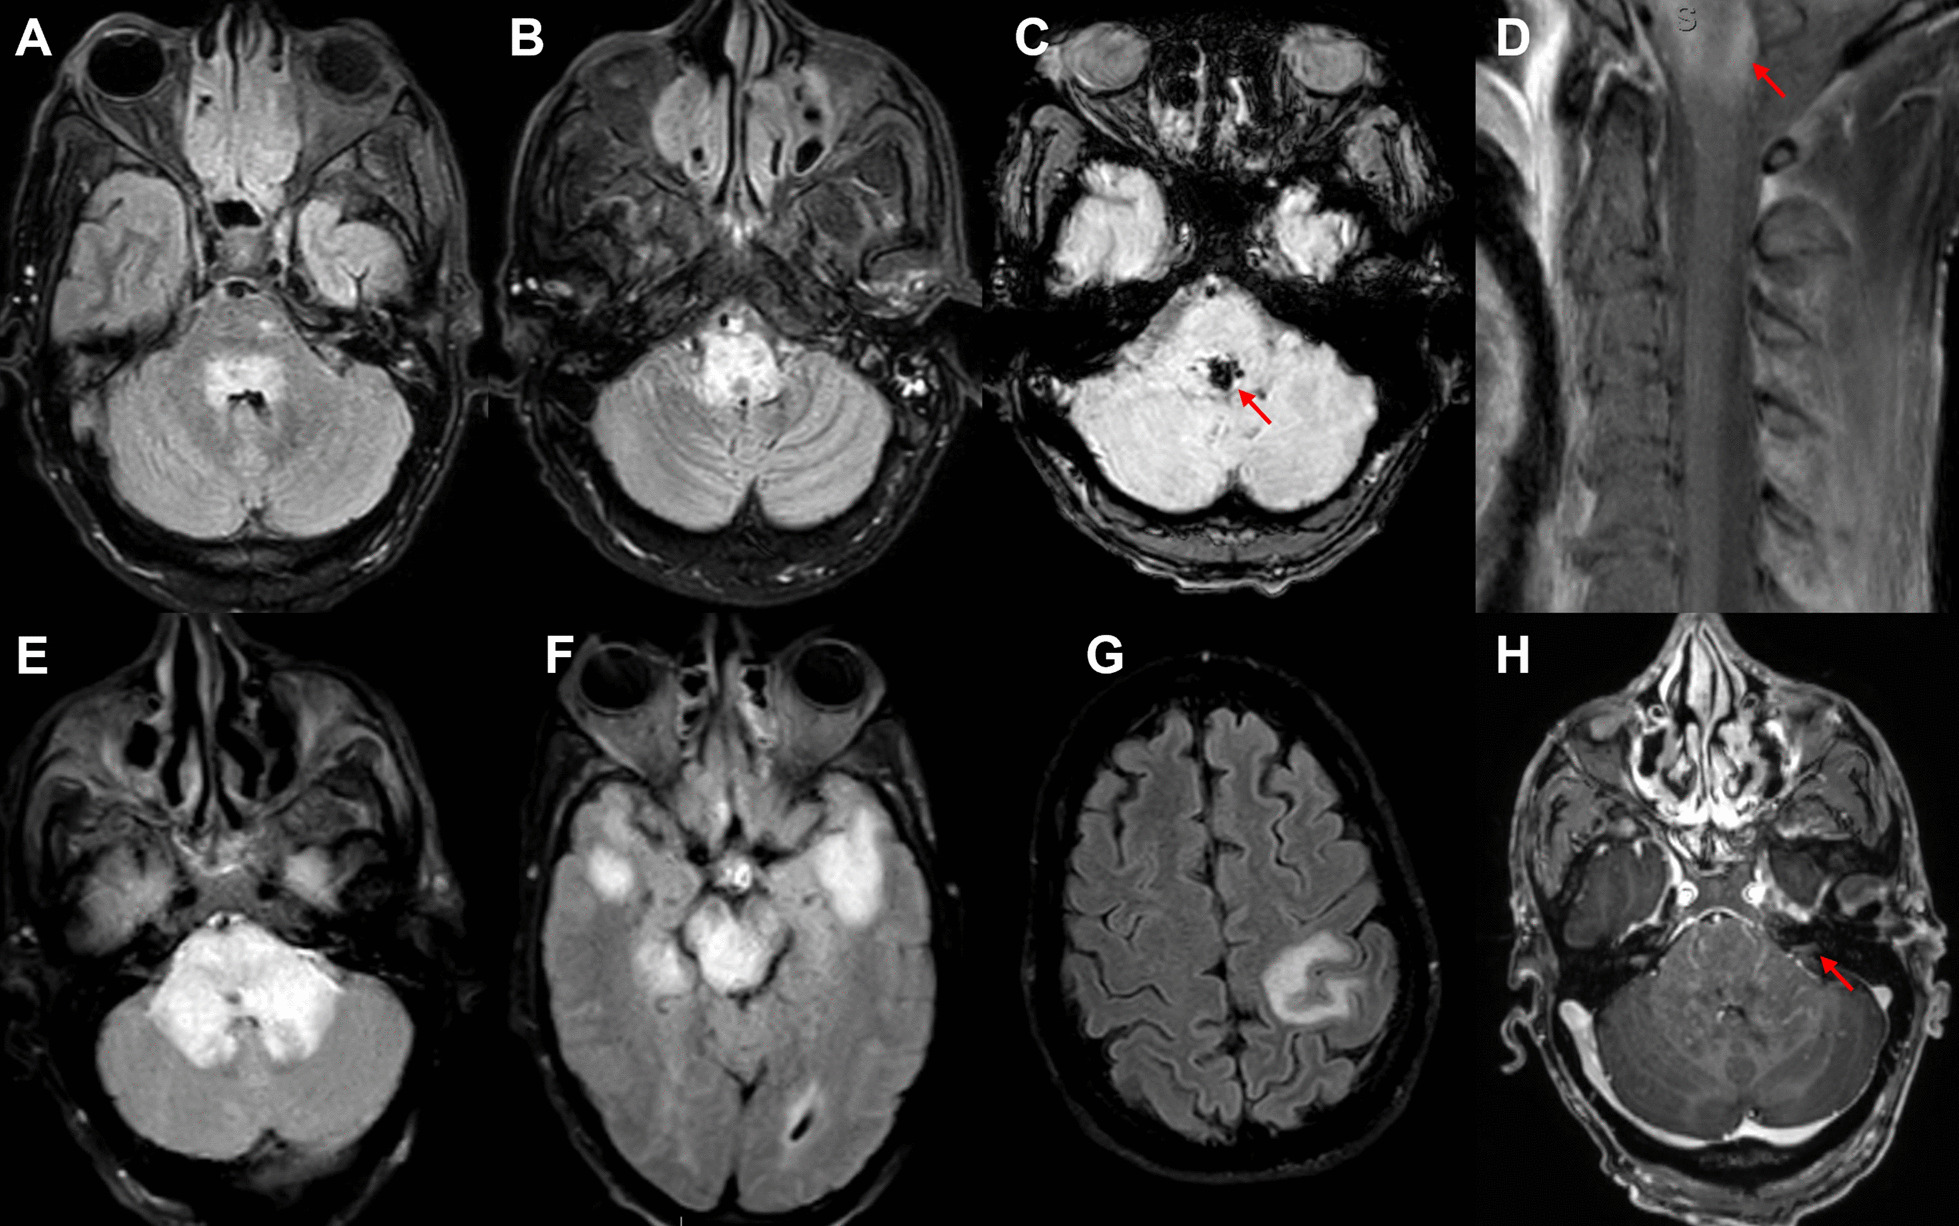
Primary CNS post-transplant lymphoproliferative disorder radiography. Initial brain MRI showing fluid attenuation inversion recovery (FLAIR) hyperintensities within the dorsal pons (A) and throughout the medulla (B) with foci of susceptibility within the pons (C). MRI of the cervical spine with T1 fat saturation sequence showing contrast enhancement of the upper cervical cord (D, arrow) with leptomeningeal enhancement. MRI one month later when the patient presented with left hemisensory symptoms showing multiple FLAIR hyperintense lesions in the pons (E), midbrain and bilateral temporal lobes (F), and left parietal lobe (G). Additionally, contrast enhancement within the middle cerebellar peduncles was seen (H)
